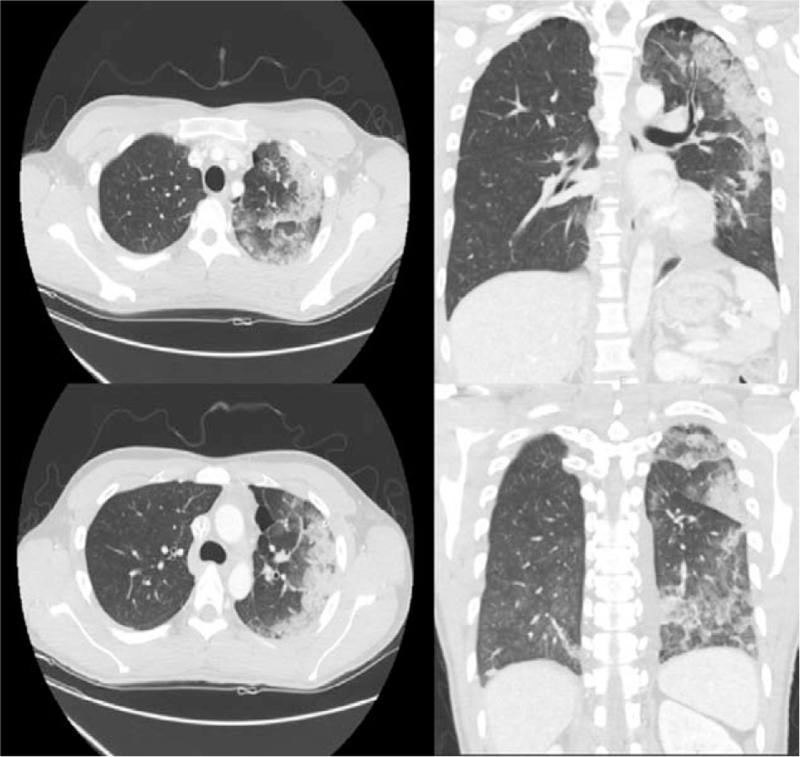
Chest computed tomography (CT) scan shows multiple irregular patchy consolidation and interlobular septal thickening in the left lung.
